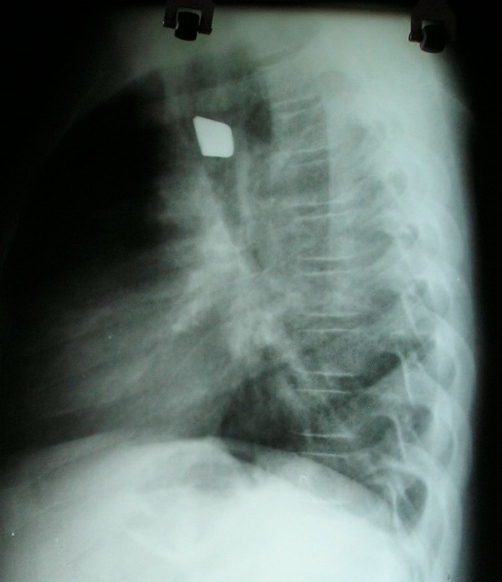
Lateral chest X-ray showing radio-opaque foreign body.
